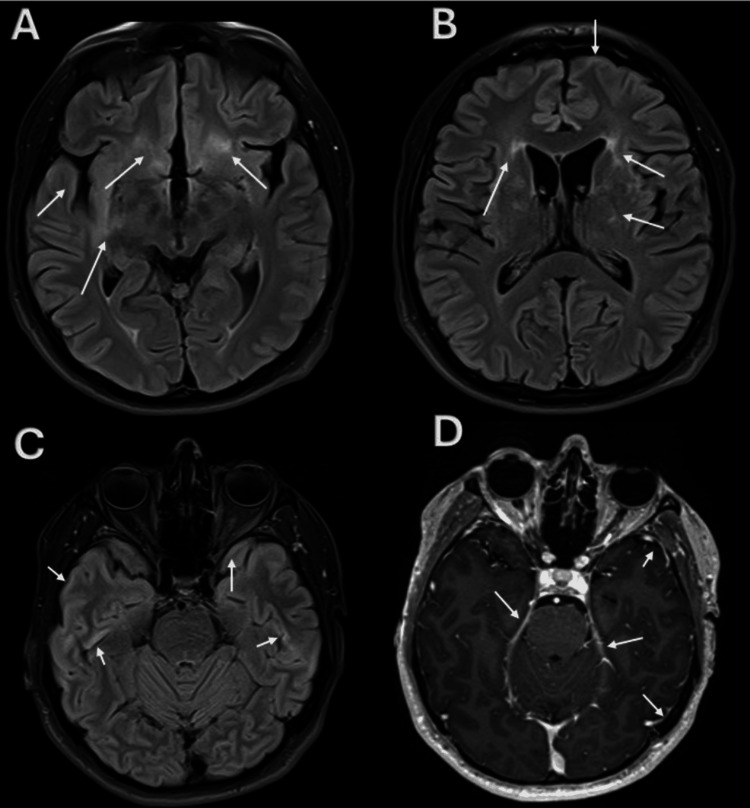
Axial magnetic resonance imaging (MRI). (A, B) Fluid-attenuated inversion recovery (FLAIR) sequence shows reduced brain volume in the temporal region and hyperintense areas in the bilateral frontotemporal region, primarily around the anterior horns of the lateral ventricles. (C) Cortical hyperintensity in the temporal lobes. (D) Axial post-contrast section showing leptomeningeal enhancement in the bilateral temporal cortex. Arrows indicate areas of pathological signal. Images correspond to the patient's initial MRI and are published with informed consent. All identifying information has been removed.
